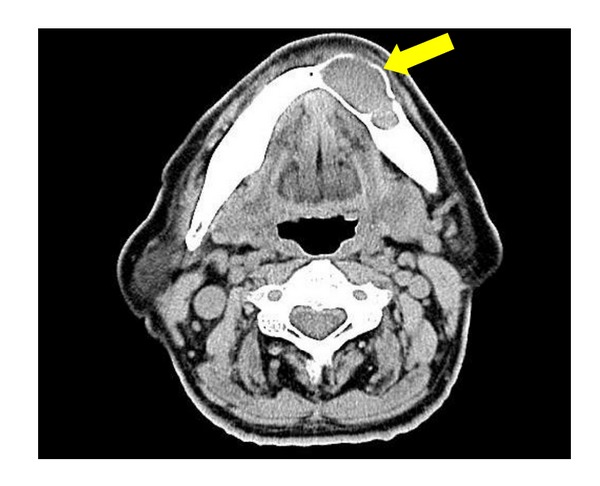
Computed tomography view. This shows thinning of peripheral bone was observed, with the bone lacking continuity in some areas.
